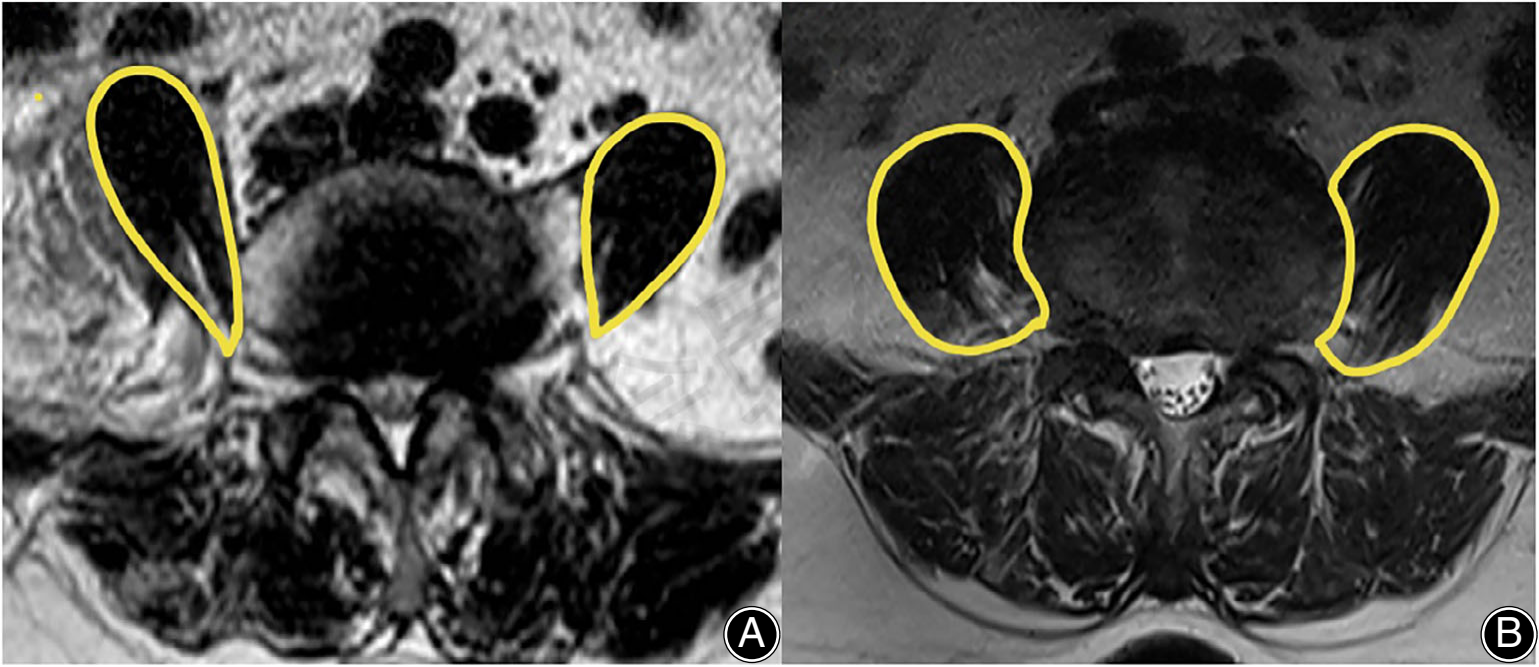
Morphology of psoas major on MRI. (A) Bilateral teardrop‐shaped psoas major; (B) Bilateral normal‐shaped psoas major.
